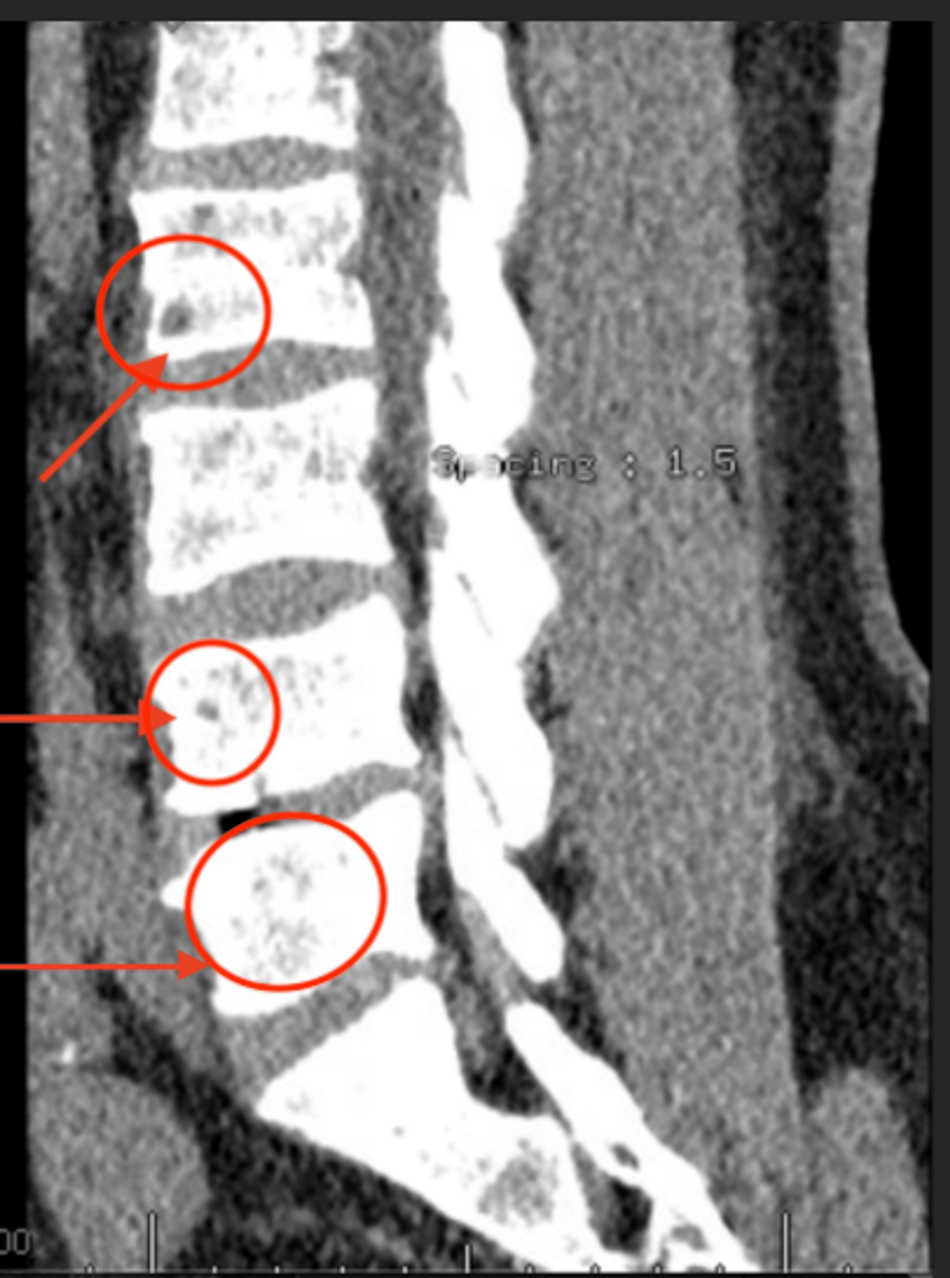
Sagittal CT of the lumbar spine showing SI joint fusion as well as multiple lytic lesions
